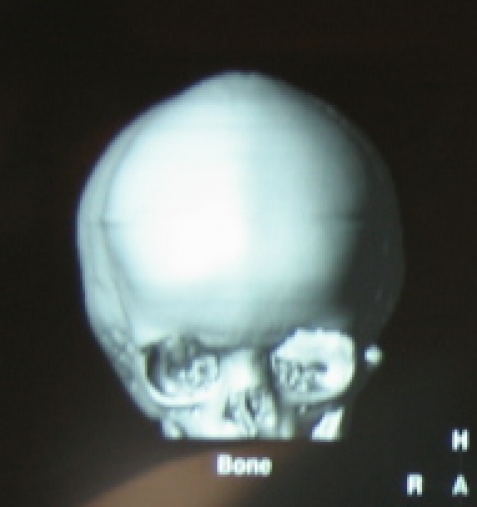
Frontal 3D CT in scaphocephaly
